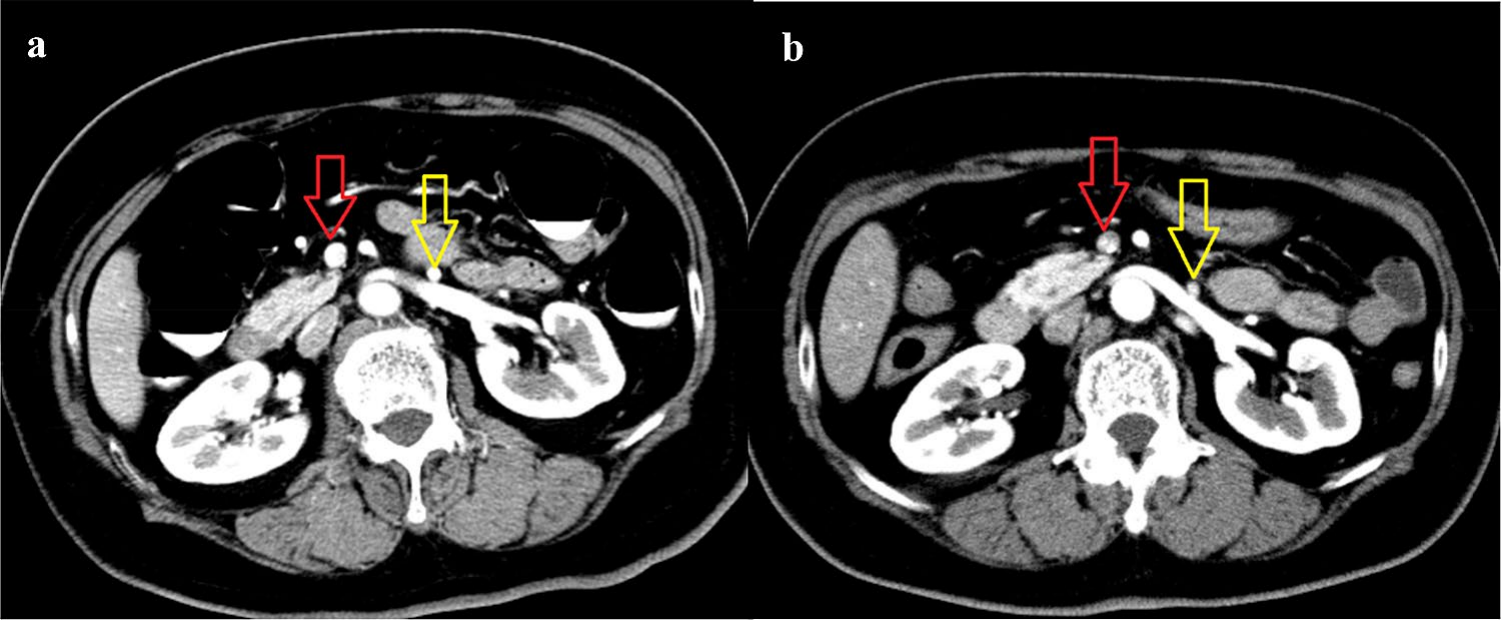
Early-phase CT (a) obtained after CTC with carbon dioxide expansion (CE-CTC), and early-phase CT (b) obtained without CTC procedures (control) from a same 63-year-old woman. The contrast enhancement effect of the SMV (red arrow) and IMV (yellow arrow) in the early-phase CT obtained after CTC with carbon dioxide expansion was higher than that obtained without CTC procedures. Dilatation of the SMV and IMV in a) was also observed
